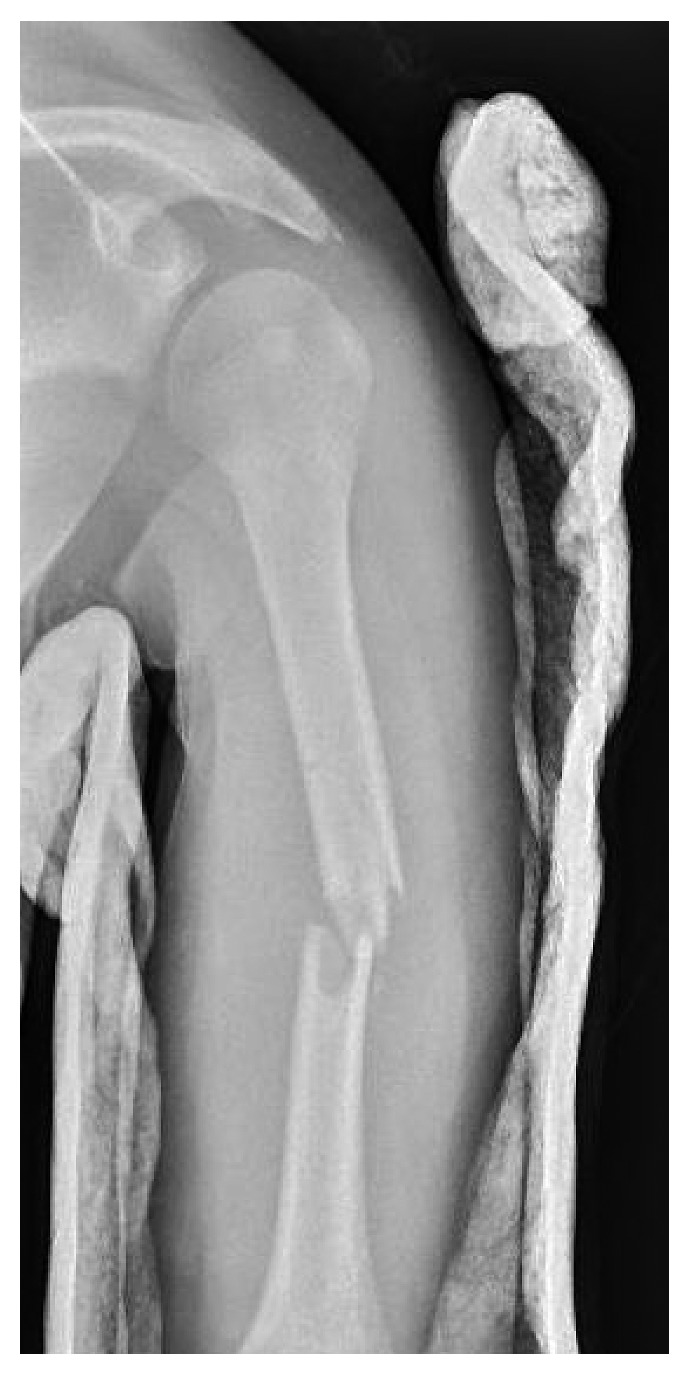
Anteroposterior radiograph following reduction and coaptation splinting showing some mild distraction but overall improvement of alignment of the humerus fracture with acceptable residual 15° varus angulation.
